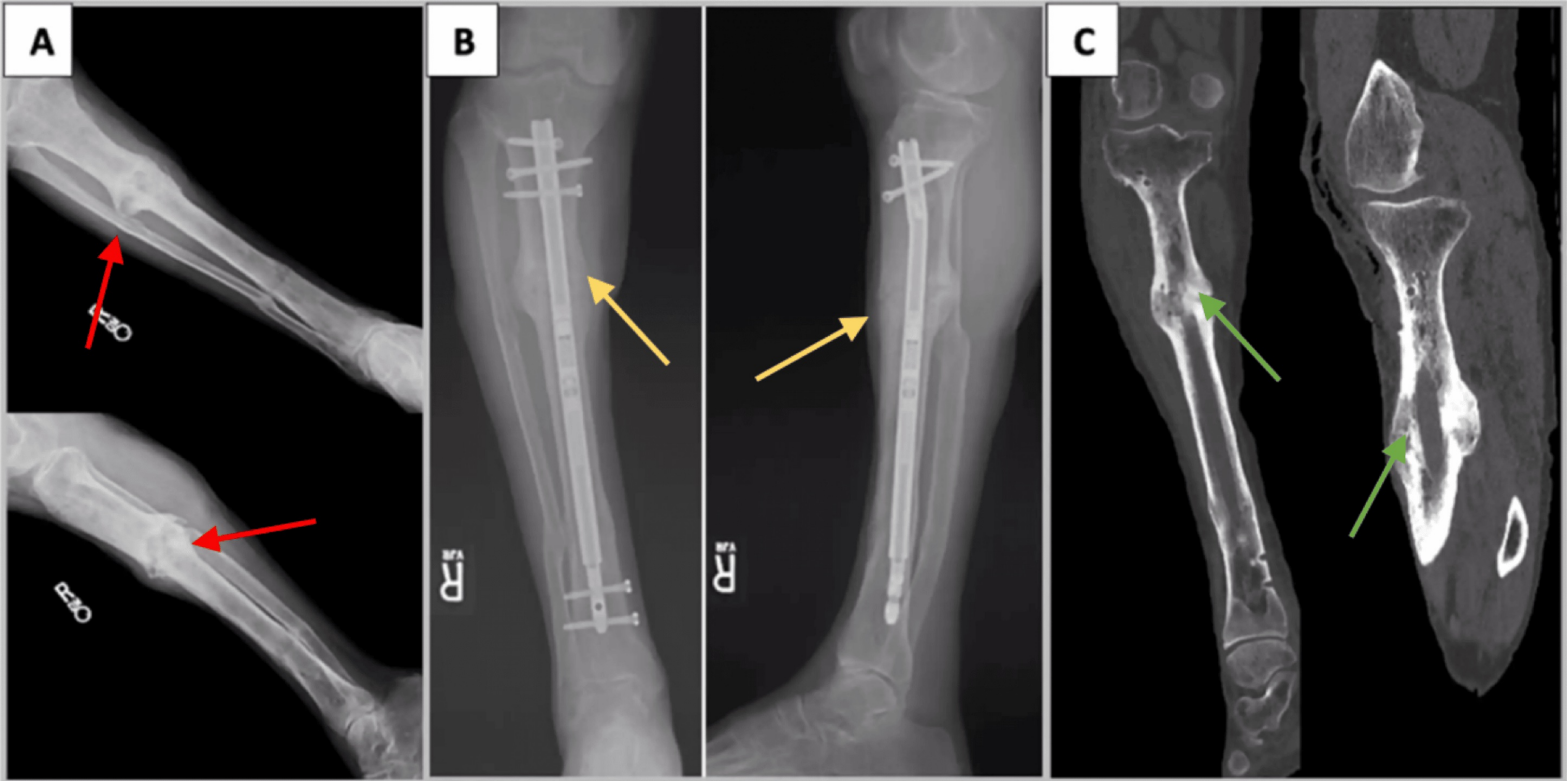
A 69-year-old male smoker with a hypertrophic tibial nonunion (case 2) A) Radiographs after failed Ilizarov external fixation treatment complicated by osteomyelitis demonstrating a persistent hypertrophic nonunion (red arrows). B) Approximately one year after exchange nailing with cortical venting and insertion of a telescoping motorized intramedullary nail in which bridging callous formation (yellow arrows) is evident. C) CT of the tibia at 18 months after modified exchanged nailing procedure and subsequent intramedullary nail removal demonstrating union (green arrows).
